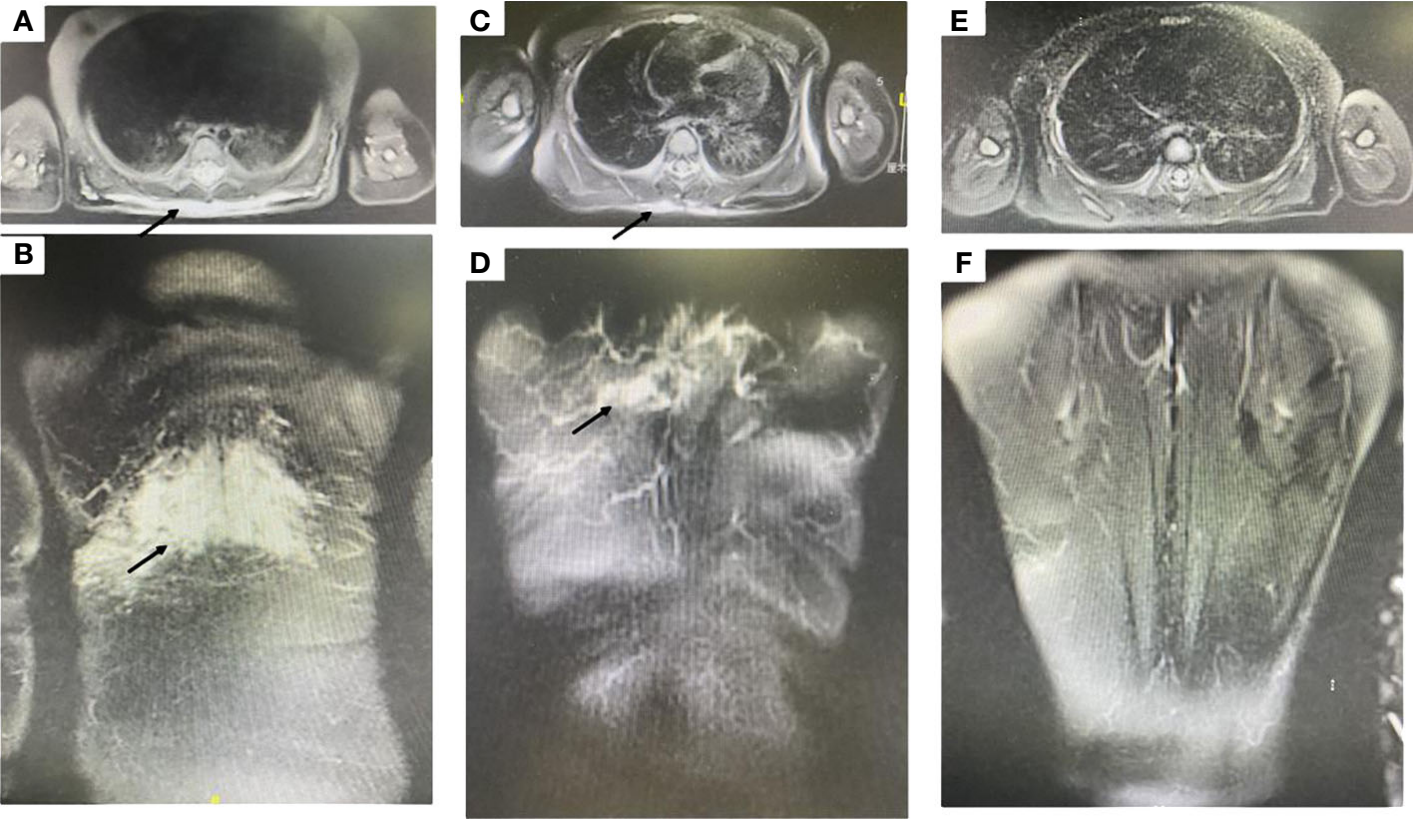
(A, B) Magnetic resonance imaging showed ill-defined margins, diffusive hyperintense signals on fat-suppressed T2 weighted images (black arrow designates the lesion). (C, D) After one year of sirolimus treatment, the lesion shrank dramatically (black arrow designates the lesion). (E, F) After two years of sirolimus treatment, the lesion regressed completely.
